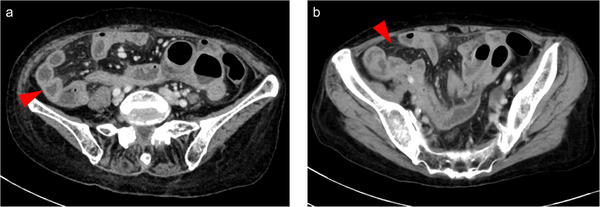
Abdominal computed tomography (CT) revealed (a) wall thickening of the small intestine with luminal fluid retention (red arrow) and (b) increased attenuation of the mesenteric fat (red arrow).
